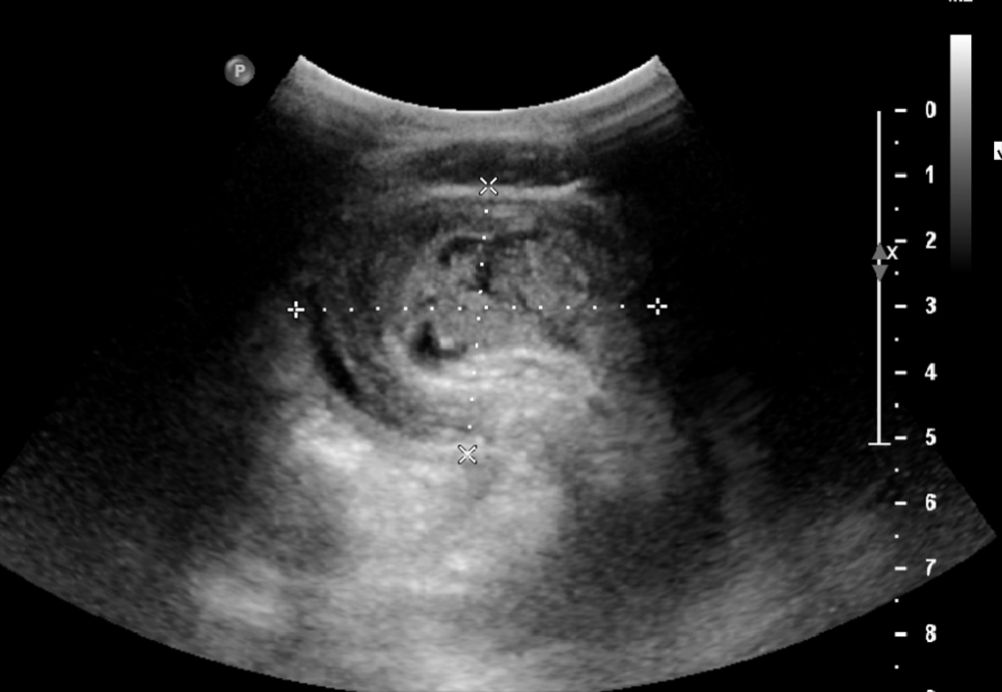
Cross-sectional ultrasound showing small bowel intussusceptionwith a “targetoid” appearance measuring 5.5 × 4.1 cm.
